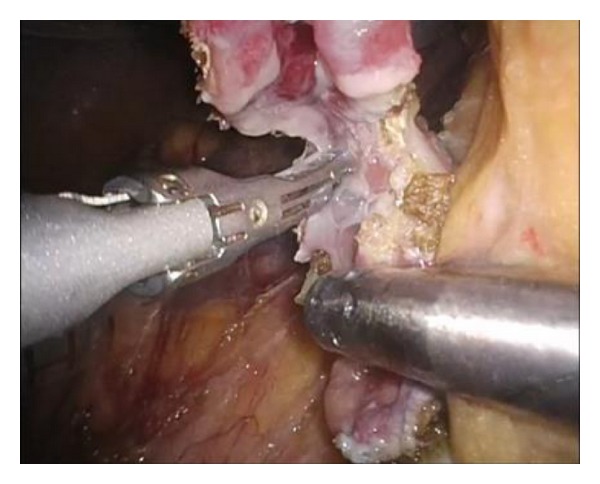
Laparoscopic view of full-thickness incision. After completion of entire circumferential seromuscular layer incision, the affected whole layer was dissected along sero-muscular layer incision circumferentially, using an ultrasonically activated device.
